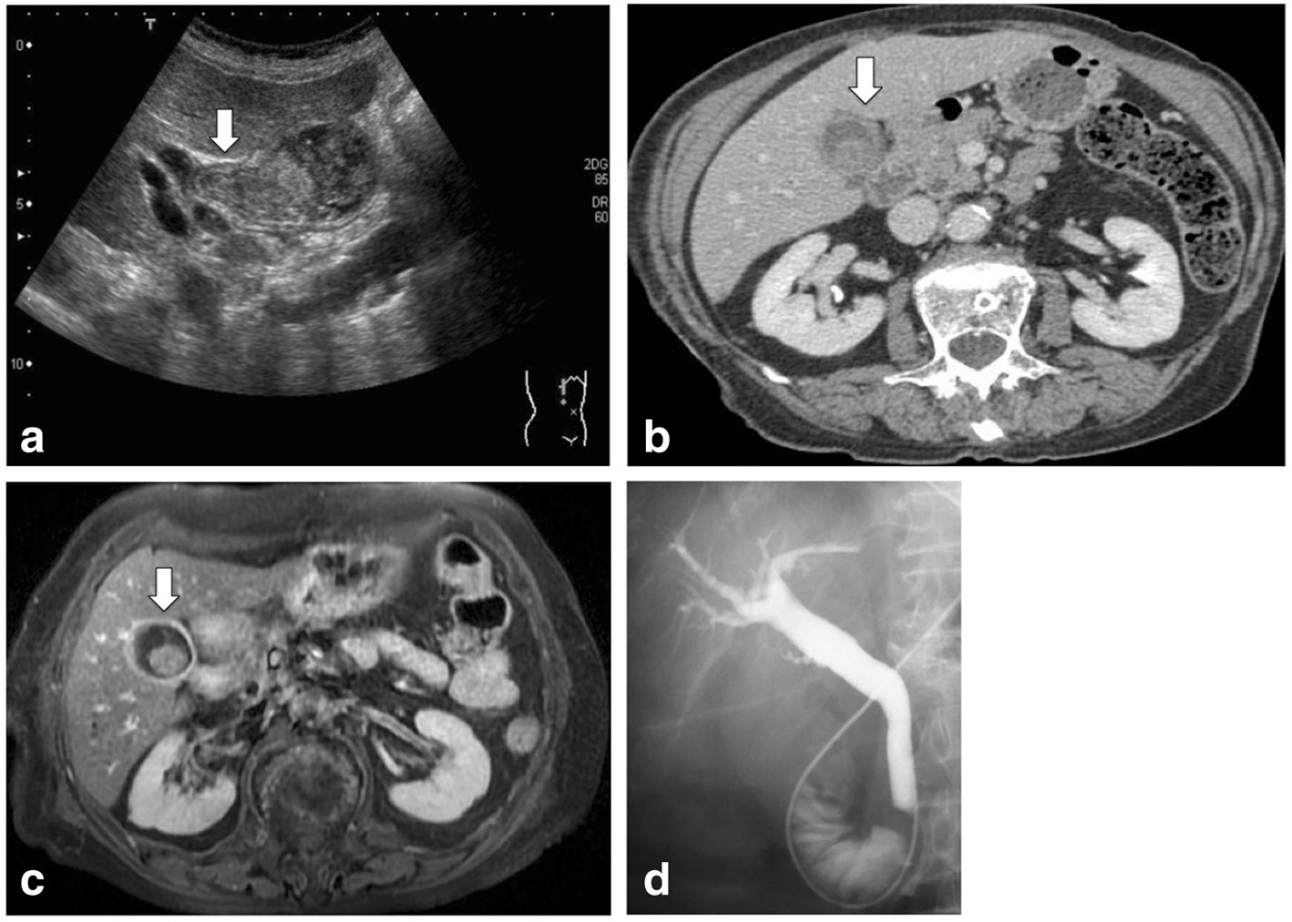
The second preoperative imaging diagnoses. (a) Ultrasonography, (b) computed tomography, and (c) magnetic resonance imaging (axial, T2-weighted image) findings. A 3.0 × 3.0-cm mass with enhancement was detected in the gallbladder; however, no lymph node enlargement or distant metastasis was observed. (d) Endoscopic retrograde cholangiography findings. Normal biliary tract without gallbladder filling was observed; however, hemobilia was recognized based on endoscopic nasal biliary drainage observations.
